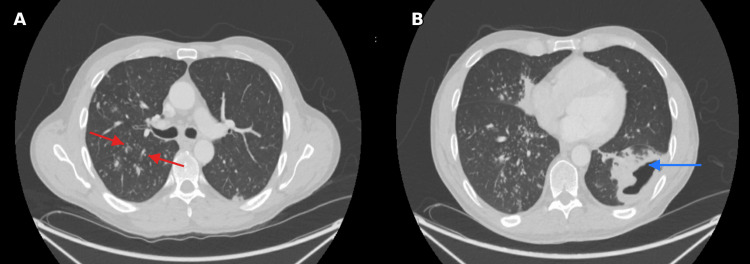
Chest computed tomography findings Axial contrast-enhanced chest computed tomography images. Panel A demonstrates reticulonodular opacities, with red arrows highlighting representative right-sided nodular opacities. Panel B demonstrates a 6.9 × 5.3 cm thick-walled cavitary opacity in the left lower lobe with an internal air-fluid level, highlighted by the blue arrow. No lymphadenopathy or pleural effusion is identified.
